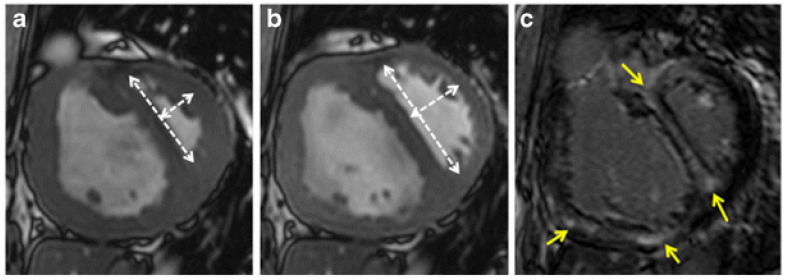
Short-axis CT images of end-diastolic cine (a), end-diastolic cine (b) and late gadolinium enhancement (LGE) (c) in a 49-year-old patient with atrial septal defect and Eisenmenger syndrome. (a) Systolic EI is 2.1. This result indicates the presence of PAH (mean PAP > 25 mmHg). (b) Diastolic EI is 2.2. This result indicates the RV systolic dysfunction (RVEF < 40%). (c) Yellow arrows represent LGE, which indicates myocardial fibrosis in RV and septum. Reprinted/adapted with permission from Ref. [11]. Copyright 2016, Springer.
